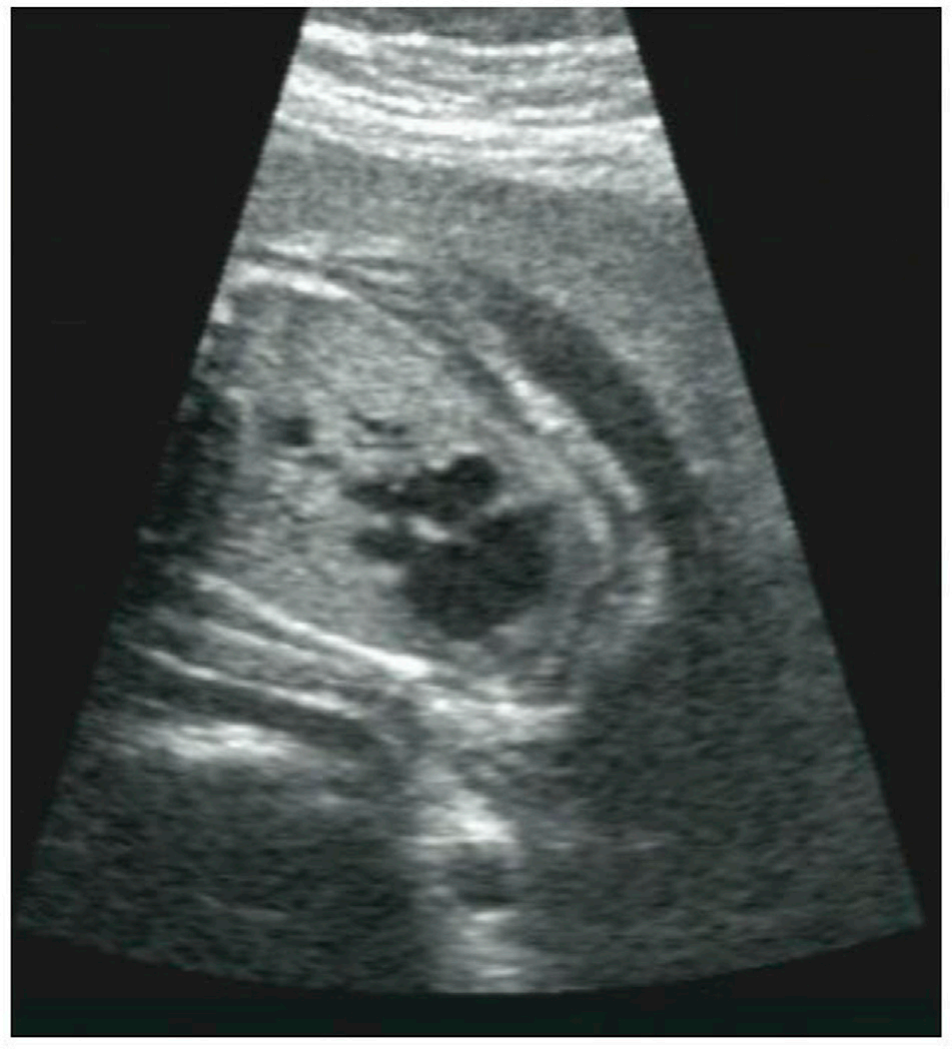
The fetus with congenital heart disease. Ultrasound scans of the fetus showed abnormal heart development, a single ventricle, pulmonary artery stenosis and situs inversus.
